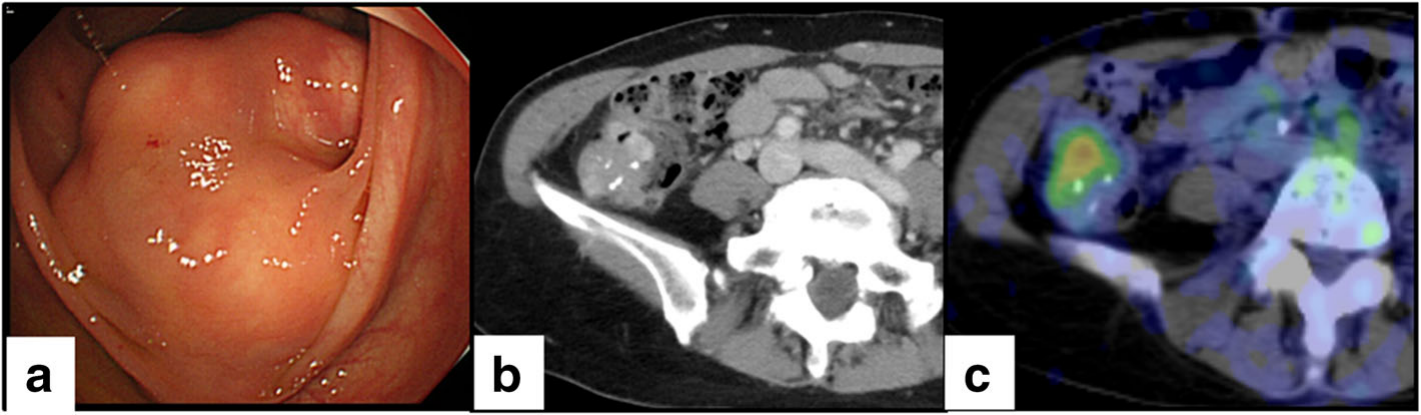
Preoperative examinations. (a) A submucosal tumor extending from the liver flexure to the ascending colon was detected by colonoscopy. (b) Computed tomography showed irregular tumor edges and highly dense areas indicative of calcification. (c) The primary tumor standardized uptake value (SUV_max_) of F-18 deoxyglucose was measured at 2.4–3.4 by positron emission tomography
